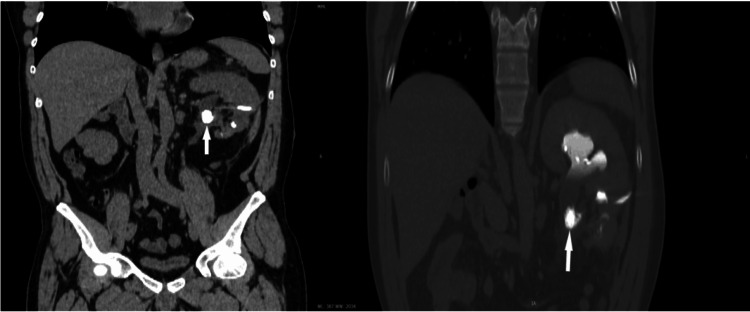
Non-enhanced CT (NECT) (a) and contrast-enhanced CT (CECT) during the urographic phase (b). The NECT scan (a) indicates the presence of a calculus within the left renal pelvis (which also happens to show a duplex collecting system, both pathways being obstructed by the calculus), clearly demarcated by the arrow. Subsequent imaging with CECT during the urographic phase (b) showcases the dilatation of the pelvicalyceal system (hydronephrosis). There are also visible partial fragments of nephrostomy tubes which should not be mistaken for calculi. This figure is the original work of the authors. Patient consent for the use of the image was obtained, as mentioned in the patient consent form (point no. 12).
